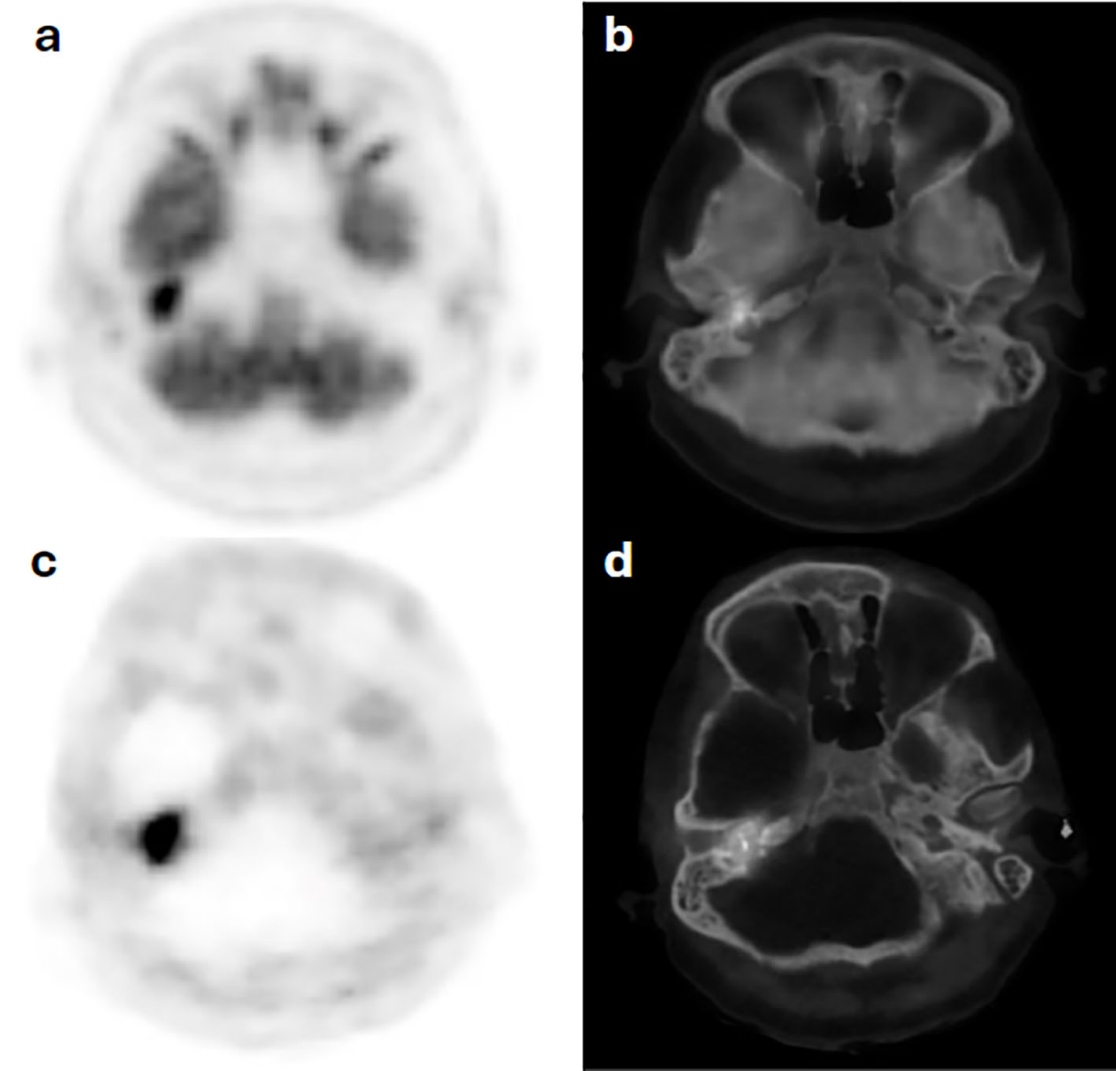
Case 4. F18-FDG PET (a) and hybrid PET/CT (b) images show increased radiotracer avidity in a tympanic bone destructive lesion on the right side with diffuse subtle avidity along the left auditory canal, which corresponds to similar uptake distribution on Ga68-DOTATATE PET (c) and PET/CT (d) scan images, not in favor of a glomus tumor on the left tympanic region.
